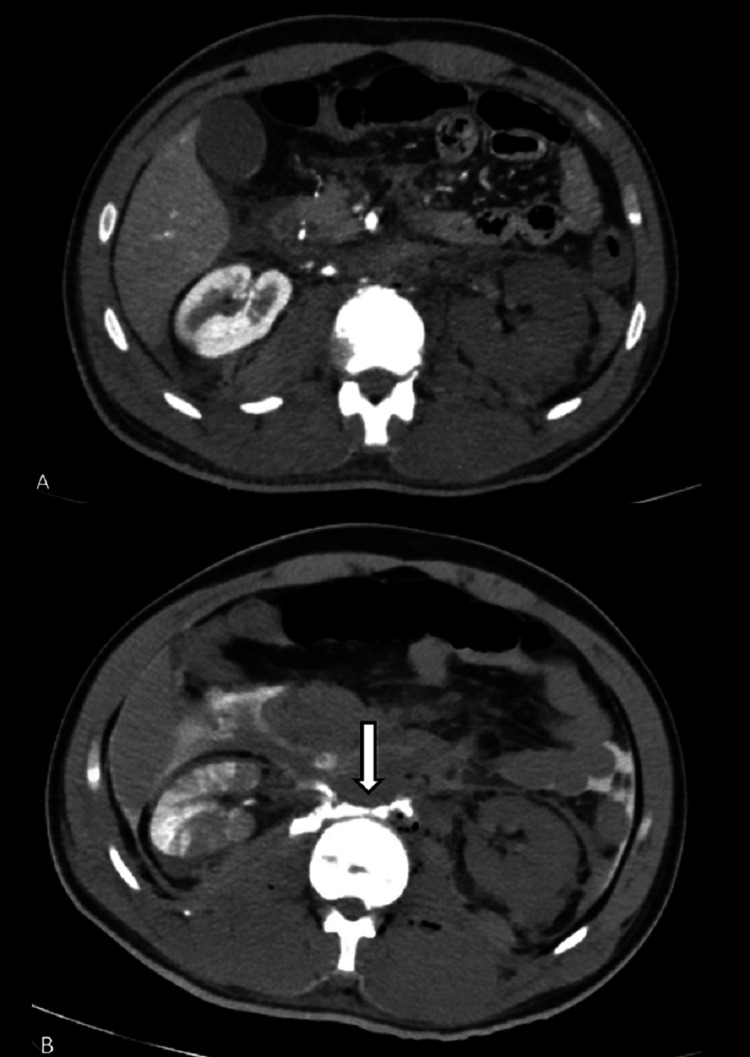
A. No significant periaortic hematoma seen on the initial multidetector computed tomography at the site of aortic occlusion. The left kidney is not showing contrast opacification due to occlusion of left renal vessels. B. On re-imaging with plain computed tomography, the contrast was selectively delivered through an embolectomy catheter placed at the upper extent of the thrombus and showed extravasation of contrast in intraperitoneal and retroperitoneal space from the aorta (arrow).
